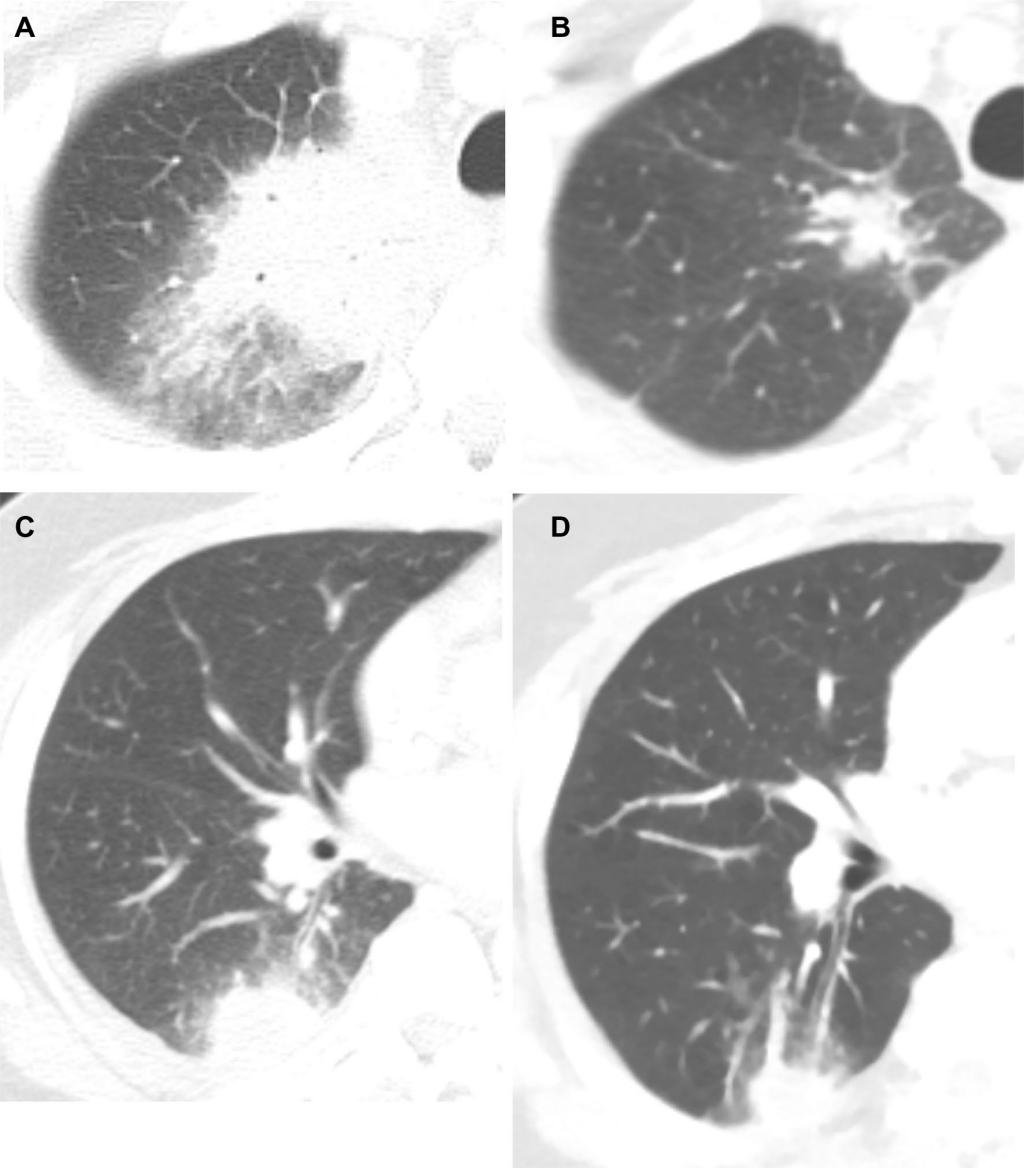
(A)–(D) Representative computed tomography findings from two patients. The first patient had a consolidation in the right upper lobe (A). A radiological response was achieved after initial treatment (B). The second patient had a nodule in the right lower lobe (C). A radiological response was not achieved (D). Invasive pulmonary aspergillosis relapsed in the second patient.
